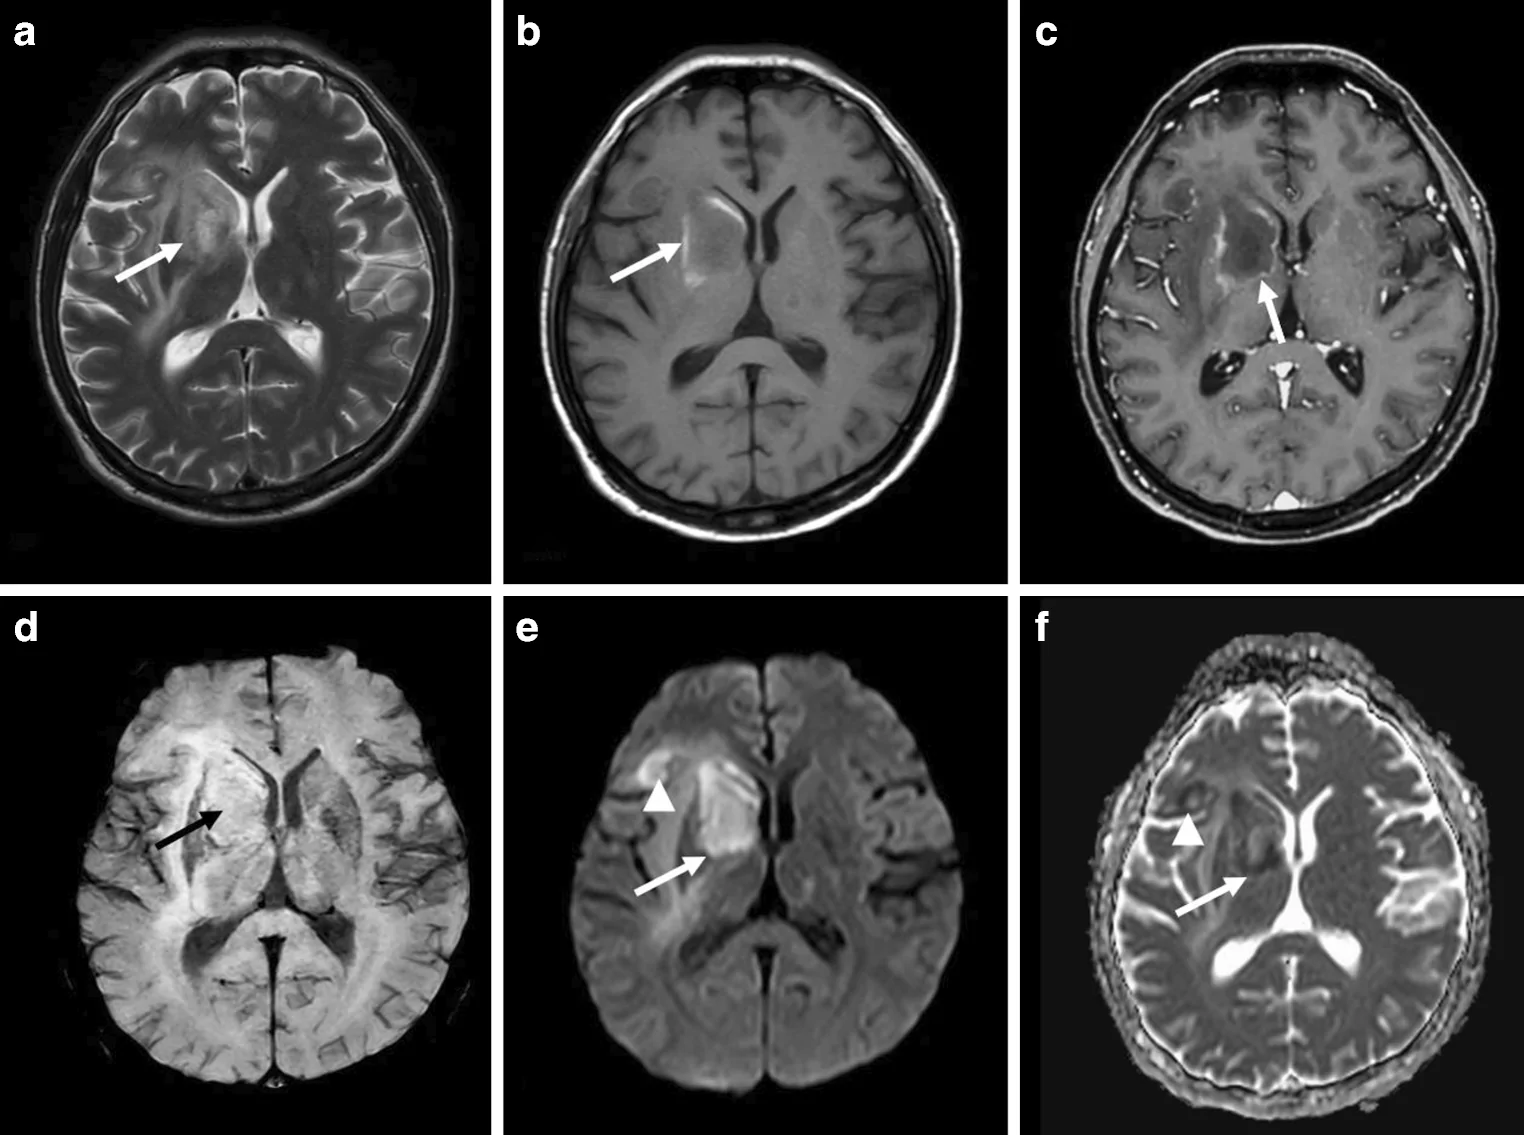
Toxoplasmosis (continued). a–f Large lesion in the right basal ganglia exhibiting concentric target sign on T2 weighted images (WI; a arrow); incomplete hyperintense rim on T1 WI (b arrow), slight enhancement (c arrow), no signal loss on SWI (d arrow) and marginal restricted diffusion (e, f arrow, arrowhead; e, f DWI, b = 1000 s/mm^2^, apparent diffusion coefficient (ADC) map)
